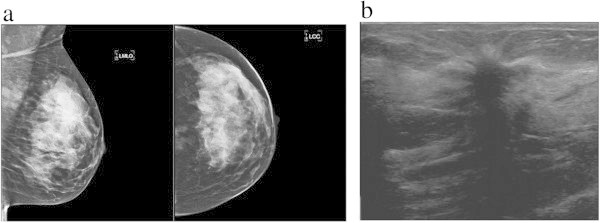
A 43 year old woman presenting with focal thickening in the left breast, finally diagnosed as ductolobular carcinoma with a ≤ 20% proportion of the lobular component. a) Mediolateral and craniocaudal mammograms of the left breast show a spiculated mass in the upper outer quadrant. b) Ultrasound shows a hypoechoic and spiculated mass, with echogenic halo at and posterior acoustic shadowing (upper outer quadrant).
